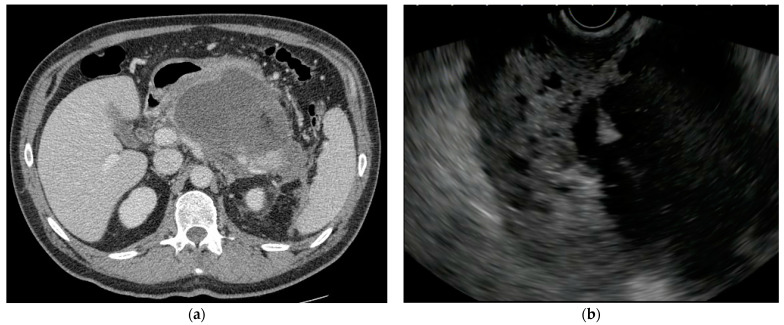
Contrast-enhanced Computed Tomography (CE-CT) (a) and EUS (b) appearance of a large WOPN located in the body-tail of the pancreas.
